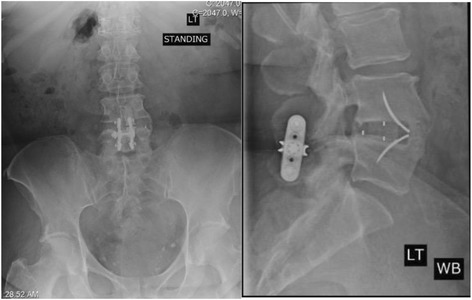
Twelve-month visit AP and lateral radiograph of subject who received 4.2mg of rhBMP2
